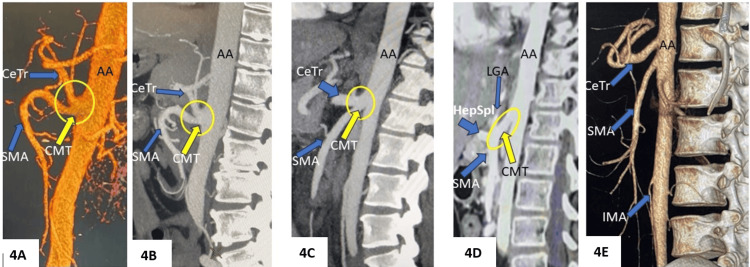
Reformatted images from CT angiography study. A-D showing celiacomesenteric trunk (CMT) in three cases. A and B represent single case, C and D represent two different cases. For comparison, image E showing a more commonly seen "normal" pattern as a separate origin of celiac trunk and SMA. CMT is encircled and indicated by a yellow arrow AA: abdominal aorta; CeTr: celiac trunk; SMA: superior mesenteric artery; HepSpl: hepatosplenic trunk; LGA: left gastric artery; IMA: inferior mesenteric artery
